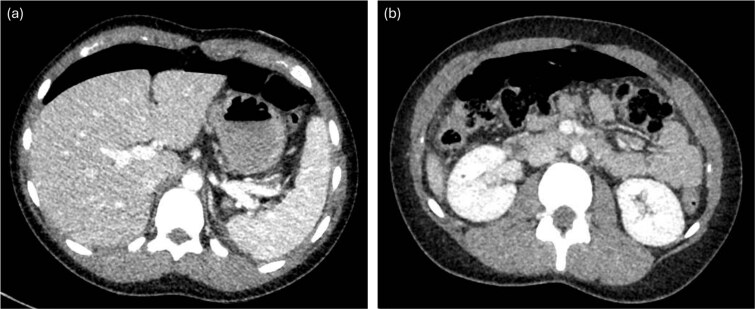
CT with intravenous contrast demonstrating large pneumoperitoneum.
